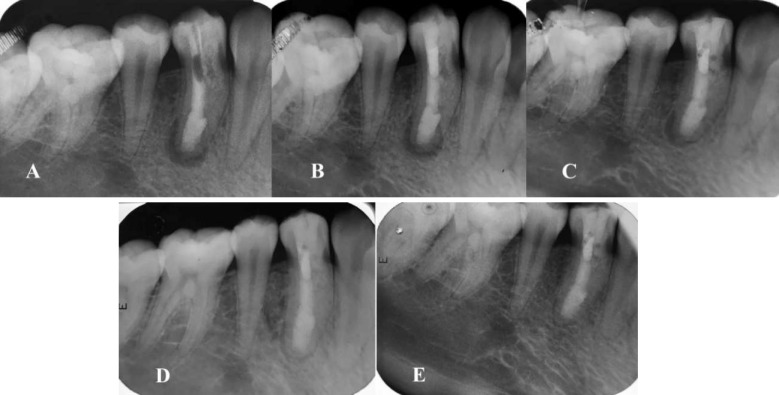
A) The ‎calcium enriched mixture ‎cement apical plug placement at the root canal and temporary restoration; B)‎ Calcium-enriched mixture ‎cement apical plug and coronal gutta-percha placement using vertical compaction technique; C) Follow-up radiograph of the tooth revealing a healing lucent lesion with diminished size 18 months after initial visit; D) Follow-up radiograph of the tooth revealing a healing lucent lesion with diminished size 24 months after initial visit; E) Periapical radiograph 36 months after the treatment
